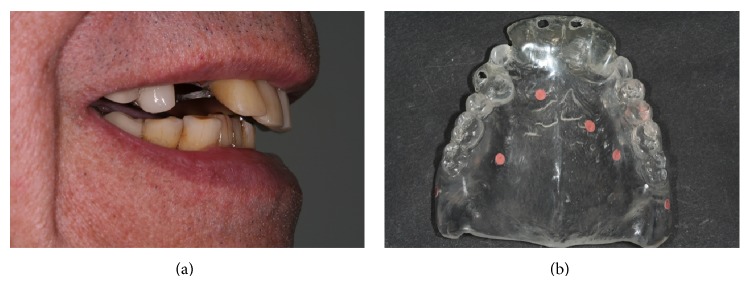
(a) Preoperative view demonstrating failing maxillary dentition. The treatment plan includes immediate implants in combination with CAD-CAM technology and their immediate loading with a prefabricated fixed prosthesis. (b) A radiographic template is realized according to the protocol for partially edentulous patient: verification windows to assess correct seating of radiographic template and radiopaque markers placed below gingival plane.
